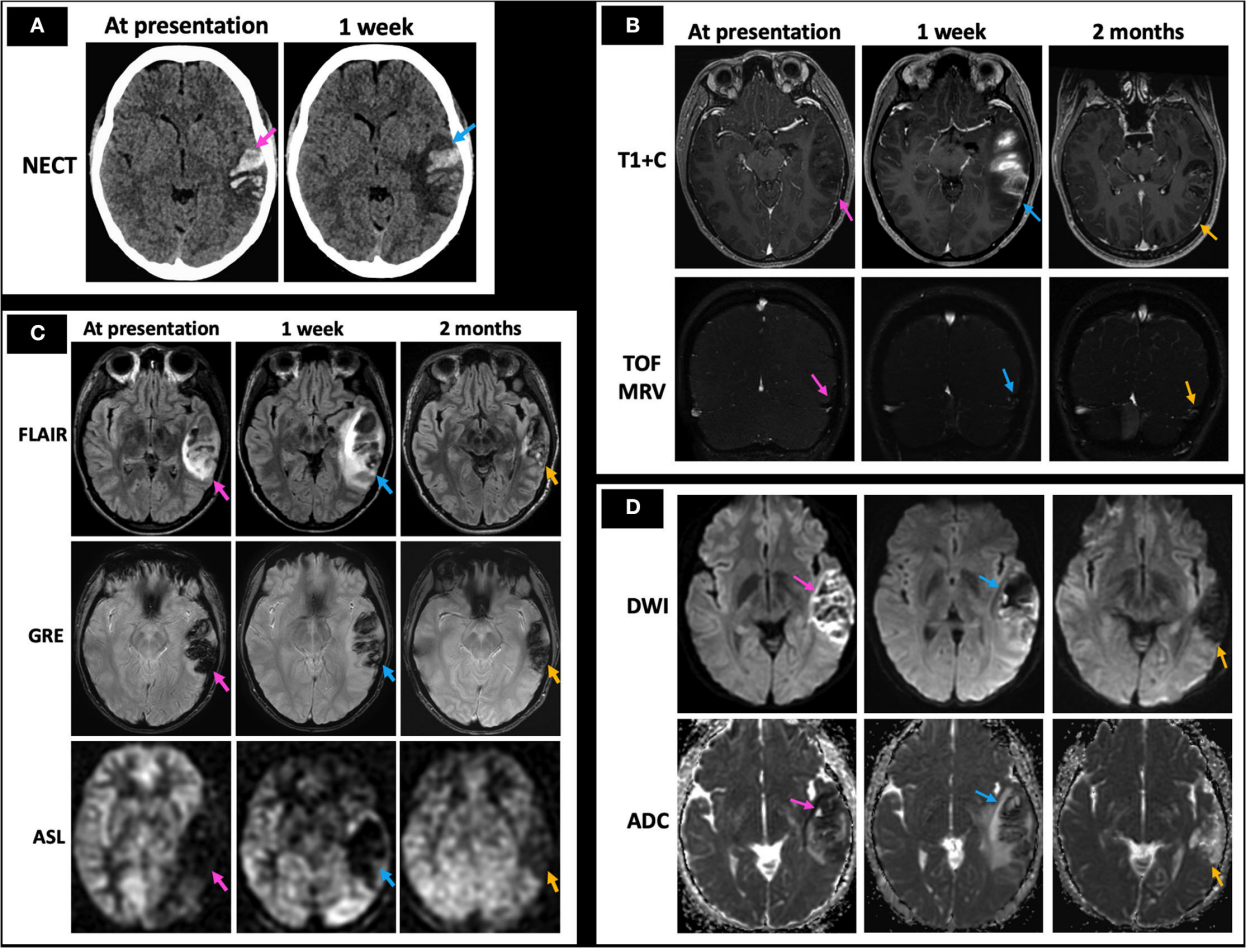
Case 6: A 26-year-old female with history of protein C deficiency, antithrombin III deficiency, and oral contraceptive use presented with difficulty reading and concentrating, as well as a left temporal headache. She was found to have non-fluent expressive and receptive aphasia on physical exam. Her initial NECT showed an acute left temporal hemorrhage (A, left image) with MRI demonstrating left vein of Labbé filling defects on T1 + C and TOF MRV (B, left column), consistent with left temporal hemorrhage secondary to a left vein of Labbé thrombosis. MRI at presentation also demonstrated surrounding FLAIR hyperintensity consistent with edema, prominent GRE hypointensity consistent with blood products, and hypoperfusion on qualitative perfusion-weighted ASL images (C, left column). She presented a week later with new right upper and lower extremity numbness, with her NECT demonstrating stable extent of the left temporal hemorrhage with increased surrounding edema (A, right image). There was also increased FLAIR hyperintensity consistent with increased edema, decreasing GRE hypointensity consistent with evolving blood products, and persistent hypoperfusion on ASL images (C, middle column) with a partially recanalized left vein of Labbé (B, middle column) and improving diffusion restriction (D, middle column). At 2 months follow-up, minimal residual filling defect in the left vein of Labbé is seen (B, right column) with markedly decreased left temporal edema as evidenced by decreased FLAIR hyperintensity and locoregional mass effect, decreased GRE hypointensity/blood products, and improved hypoperfusion (C, right column) along with resolution of diffusion restriction (D, right column). In (A–D), the pink arrows point to the imaging findings at presentation, blue arrows point to findings at 1 week, and yellow arrows point to findings at 2 months. NECT, non-contrast CT; T1 + C, post-contrast T1-weighted images; TOF MRV, time of flight MR venogram.
